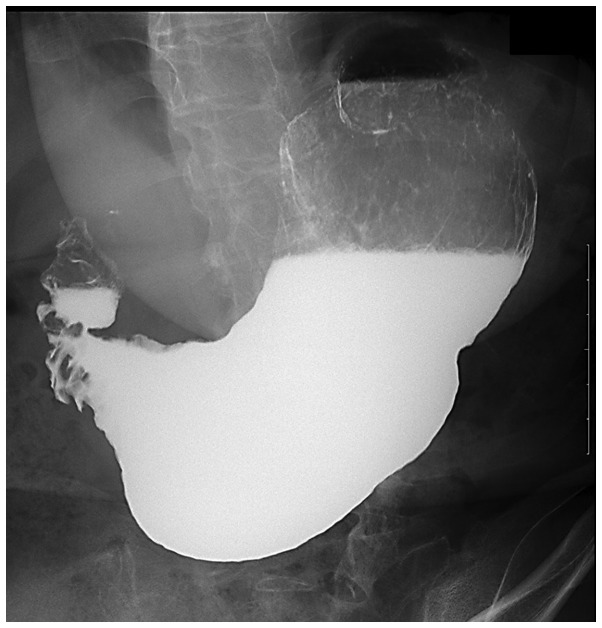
Stomach X-ray of the patient, indicating poor distension of the gastric body.
